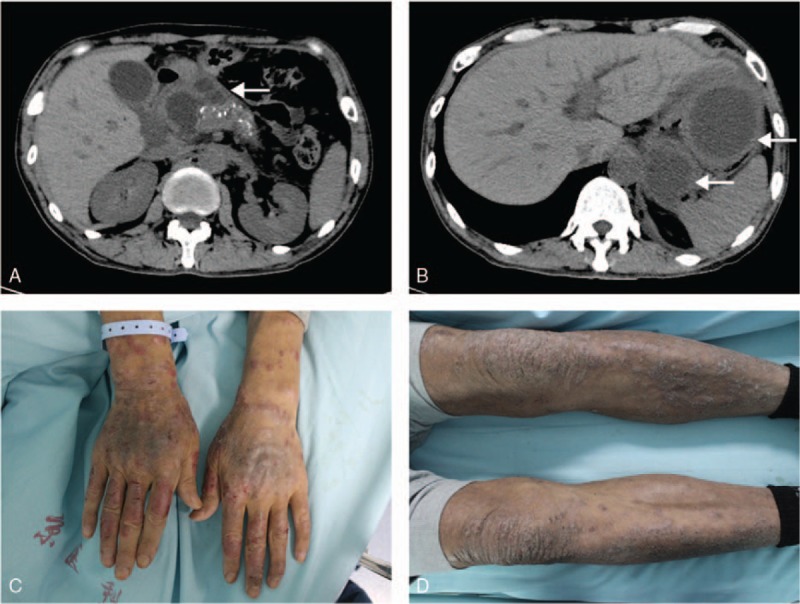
Results of abdominal CT and subcutaneous nodules 3 weeks after admission. A, CT scan shows the enlarged multiple cystic lesions in the head of the pancreas, the biggest 3.1 × 2.8 cm. B, CT scan reveals an 8.1 × 6.6 cm cystic lesion and a 5.2 × 4.1 cm cystic lesion next to the tail of the pancreas. C and D, erythematous, unclearly boundary, subcutaneous nodules of hands and lower limbs. CT = computed tomography.
